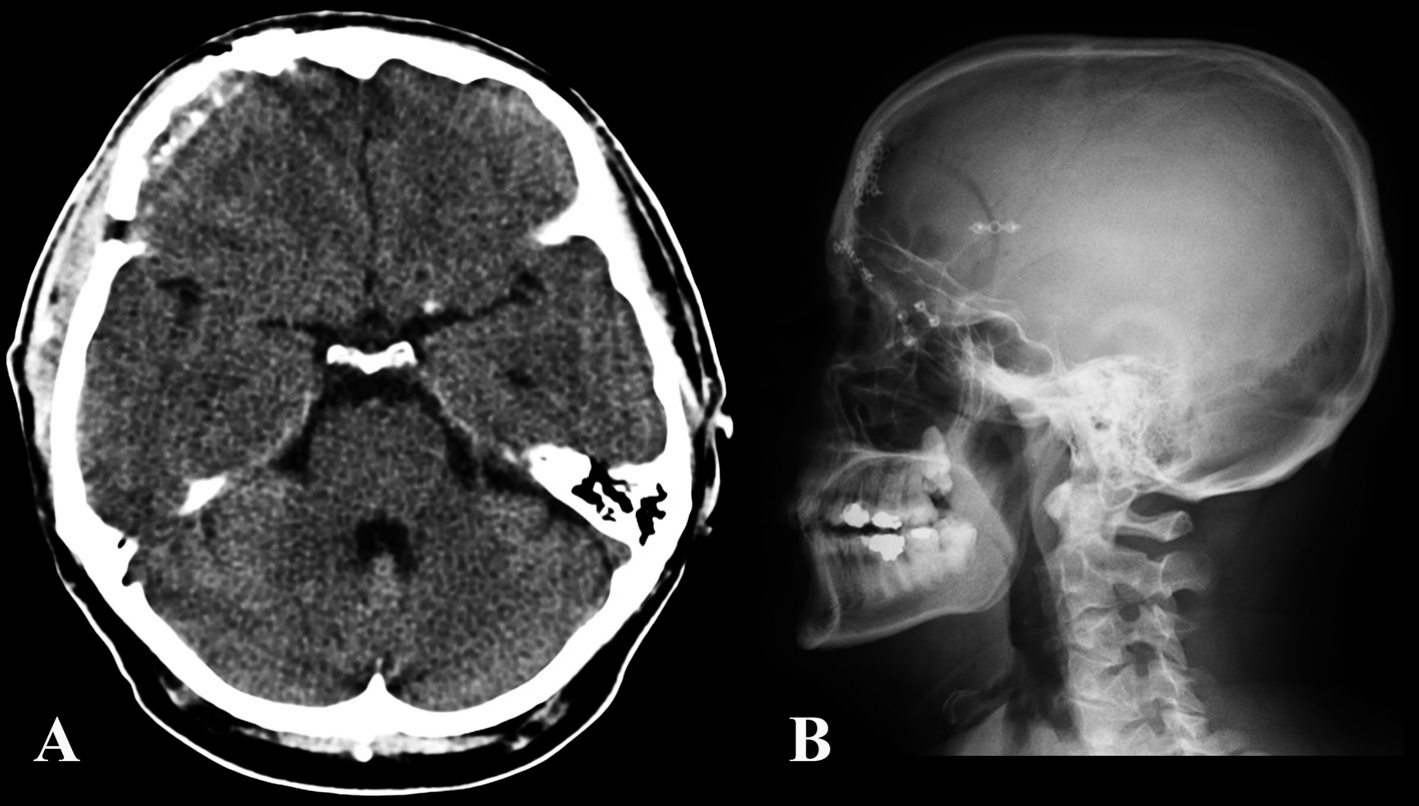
Post-operative CT (A) and skull x-plain film (B) showing the craniotomy and bony reconstruction.
